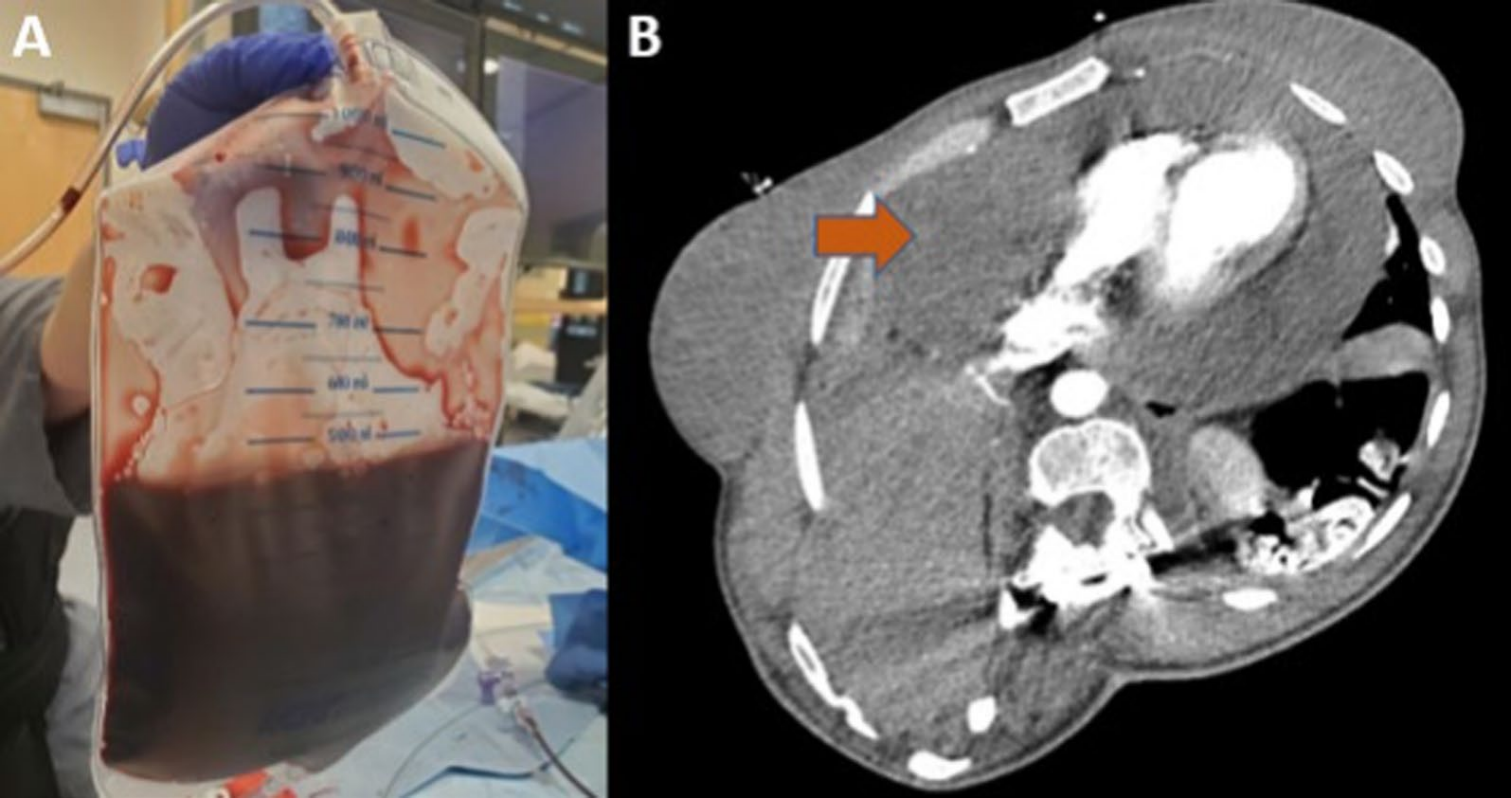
Case #1; A: Serosanguinous pericardial fluid B: Axial section from CT scan of the chest showing a large mass (orange arrow) in the anterior mediastinum along the right heart border
